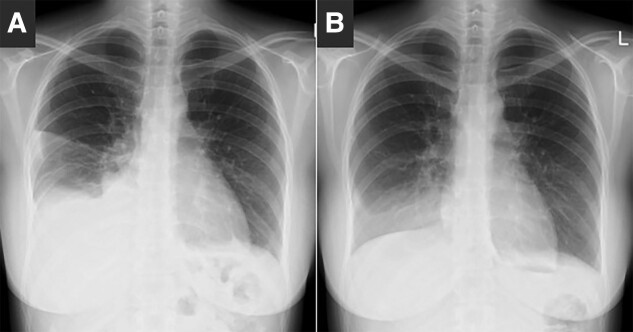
Chest x-ray. A, Bilateral right-dominant pleural effusion at presentation. B, Remarkably improved pleural effusion three days after cabergoline administration.
